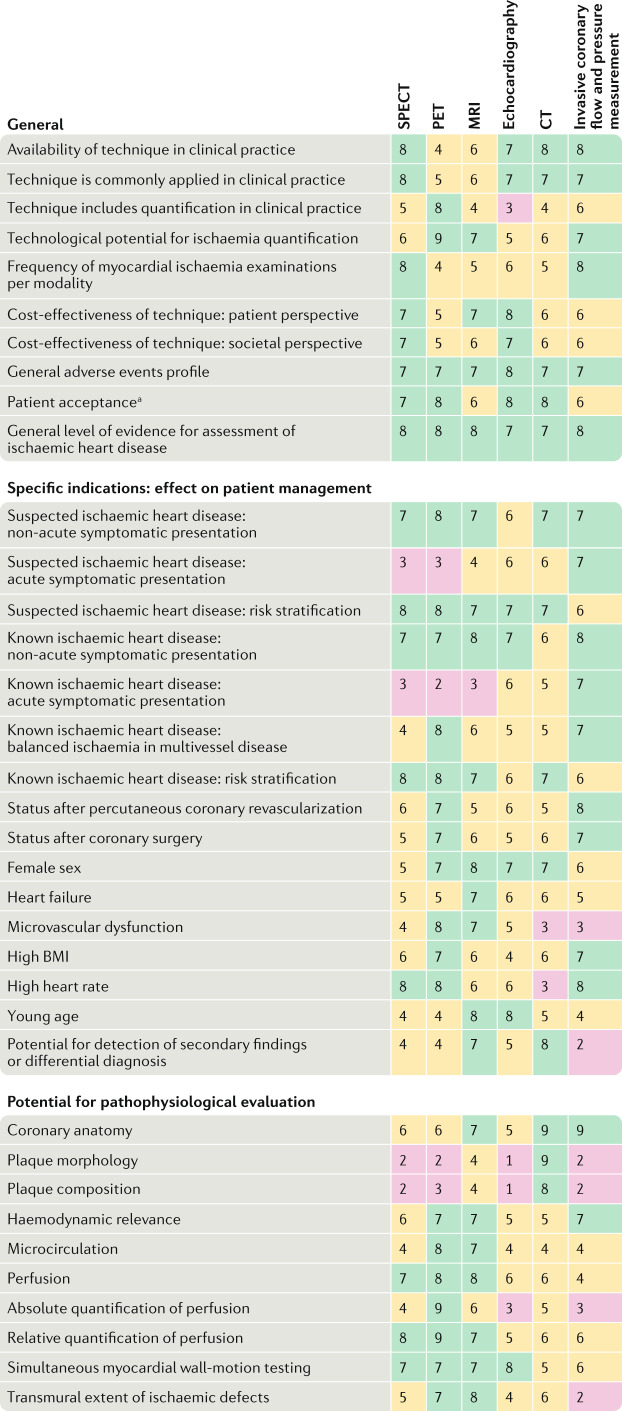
Clinical characteristics and appropriateness of myocardial ischaemia assessment tools for different patient scenarios. Consensus ratings were on a scale of 1–9, with 1–3 being inappropriate, 4–6 being uncertain and 7–9 being appropriate. A total of 16 investigators participated. A Delphi clinical consensus process was used, with ratings by eight participants (four cardiologists, two radiologists, one nuclear medicine physician and one methodologist). A separate development team of eight investigators (one dual cardiologist–radiologist, two radiologists and five methodologists) defined the questions and categories shown in the table but did not participate in the clinical appropriateness rating. ^a^Studies discussed during the meeting showed higher patient acceptance for CT than for SPECT, MRI or invasive testing^186–188^.
